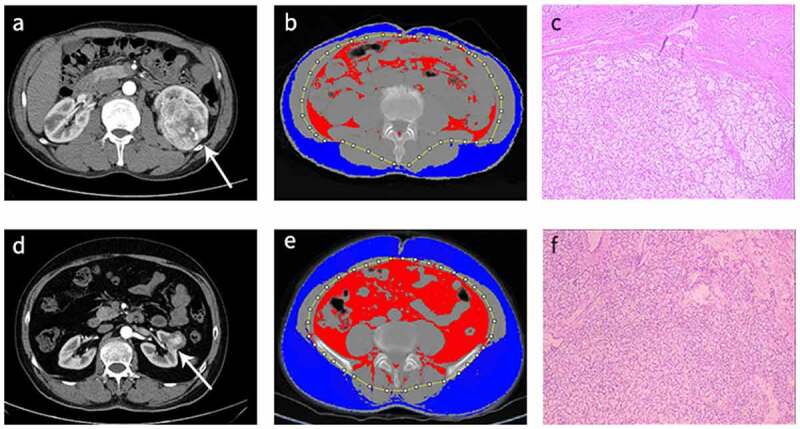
Representative preoperative images of ccRCC patients in low T-stage. (a-c) Low T-stage ccRCC patient with short-term postoperative complications in a 50-year-old man. (d-f) Low T-stage ccRCC patient without short-term postoperative complications in a 58-year-old man. (a, d) Contrast-enhanced axial CT scan images showing tumors in the left renal (white arrow). (b, e) CT images (blue areas represented regions which are subcutaneous fat, and red areas represent regions which are visceral fat) with relative visceral fat area (rVFA) of 30.2% (b) and 39.0% (e). (c, f) Pathology of the tumors showing low T-stage ccRCC.
